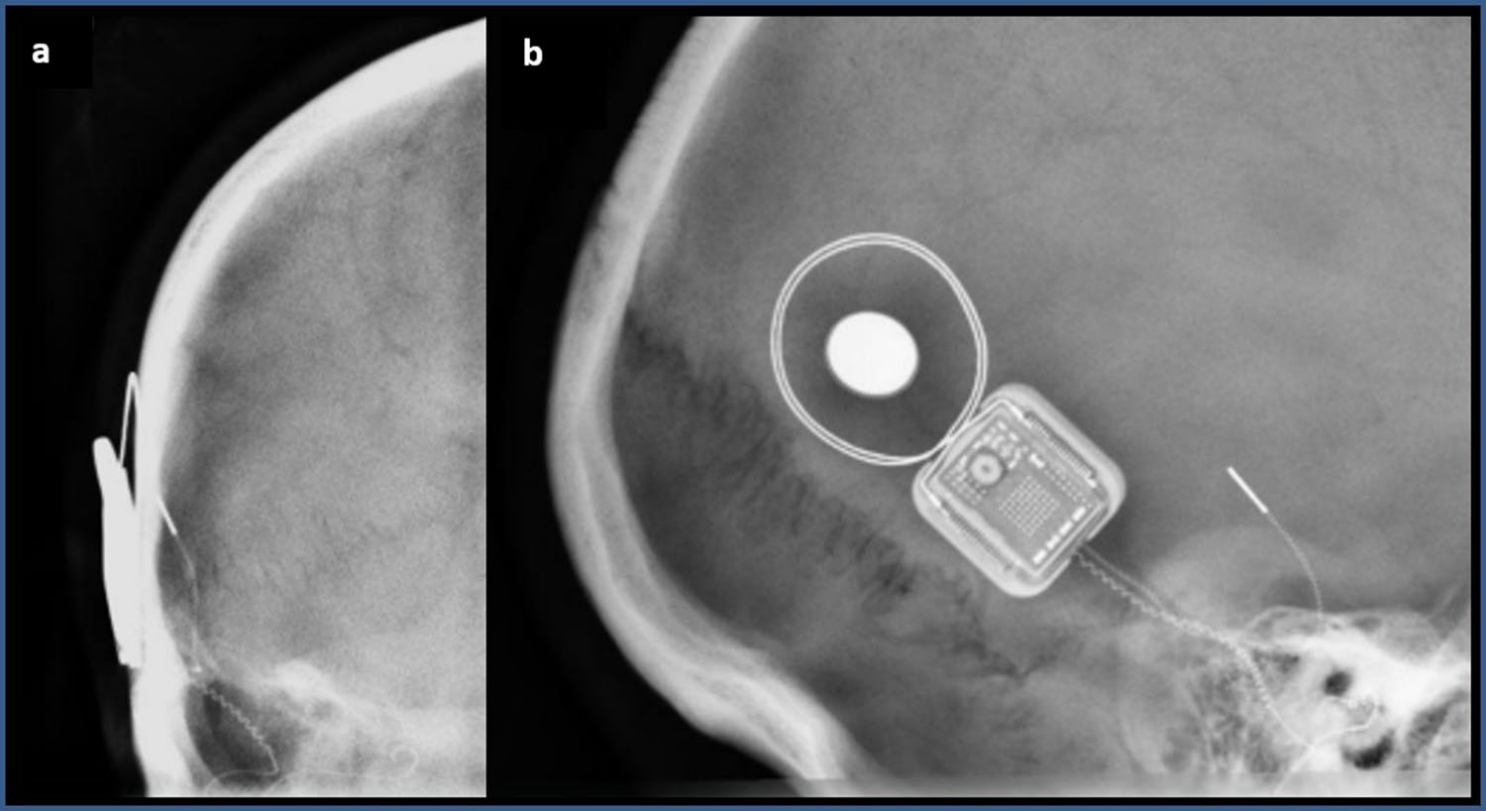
Radiographs of suspected and later confirmed magnet dislocation. a Radiograph of the skull anterior–posterior to show the case tangentially. The tilt of the magnet can be seen in the image. b Skull X-ray laterally, looking at the case from above. An off-center position of the magnet in the coil area can be seen
